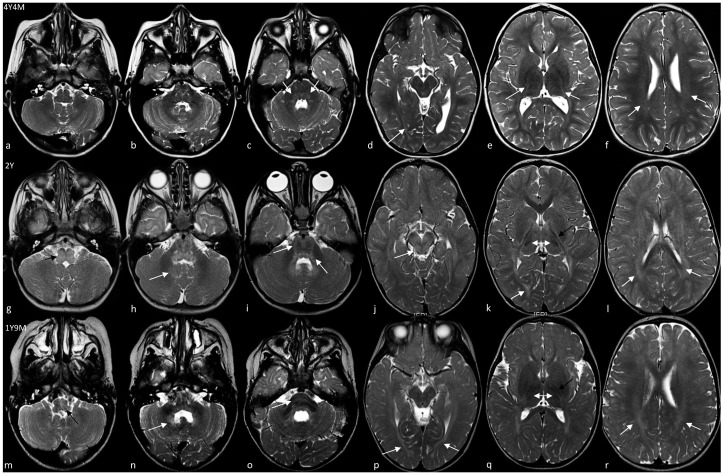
T2-weighted axial brain MRI of patient 1 (a–f), 2 (g–l), and 3 (m–r). In patient 1, medulla (a), dentate nuclei (b), and (d) midbrain are spared while mild hyperintensity is observed in dorsal pons (white arrows in c), optic radiations (white arrow in d), PLIC (white arrows in e) and periventricular white matter (white arrows in f). Patients 2 and 3 showed a more similar pattern of anomalies, with a high signal of the medulla (black arrow in g and m), hilus of the dentate nuclei (white arrow in h and n), pons (white arrow in i and o), optic radiations (white arrow in k and p) and periventricular white matter (white arrows in l and r). Please also note that the midbrain is hyperintense only in patient 2 (white arrow in j), that PLIC has a typical tram track appearance (black arrow in k and q), and that the dorsomedial thalami are hyperintense compared to the ventrolateral part (double white arrows in k and q).
